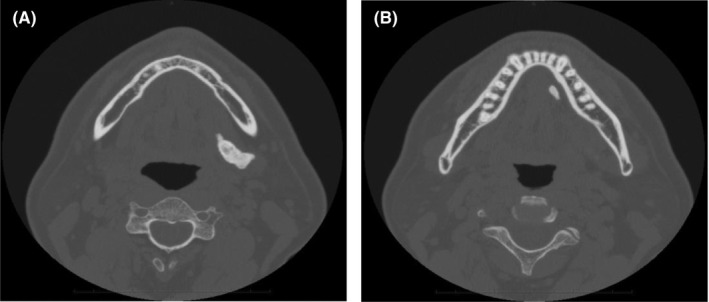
Axial computed tomography images show the left anterior and posterior stones. The posterior (A, 27 mm) and anterior (B, 9.4 mm) stones are visible
